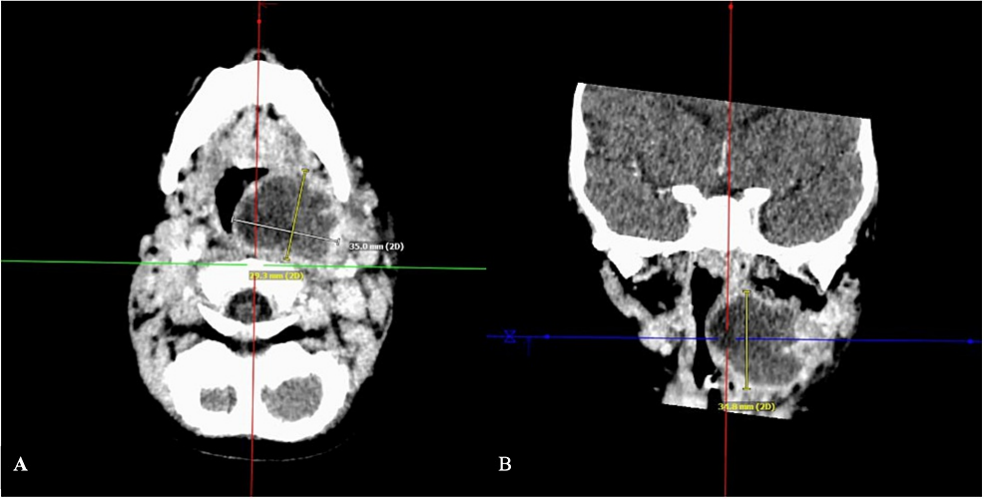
Contrasted computed tomography findings (A) axial view and (B) coronal view demonstrating a large low-attenuation fluid collection within the left para- and retropharyngeal space, resulting in airway narrowing.
